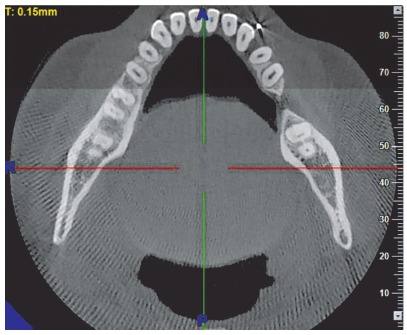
11 × 5-cm CBCT section of the mandible.
